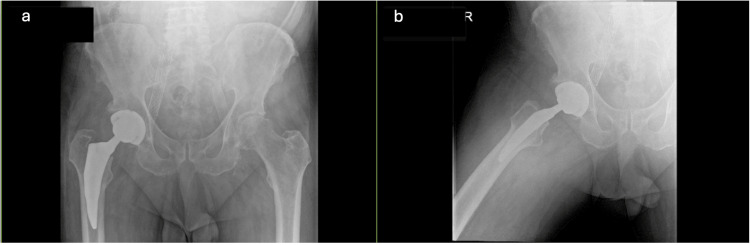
Postoperative anteroposterior (a) and lateral (b) view X-rays of the pelvis showing good prosthetic placement without evidence of malposition or loosening.
